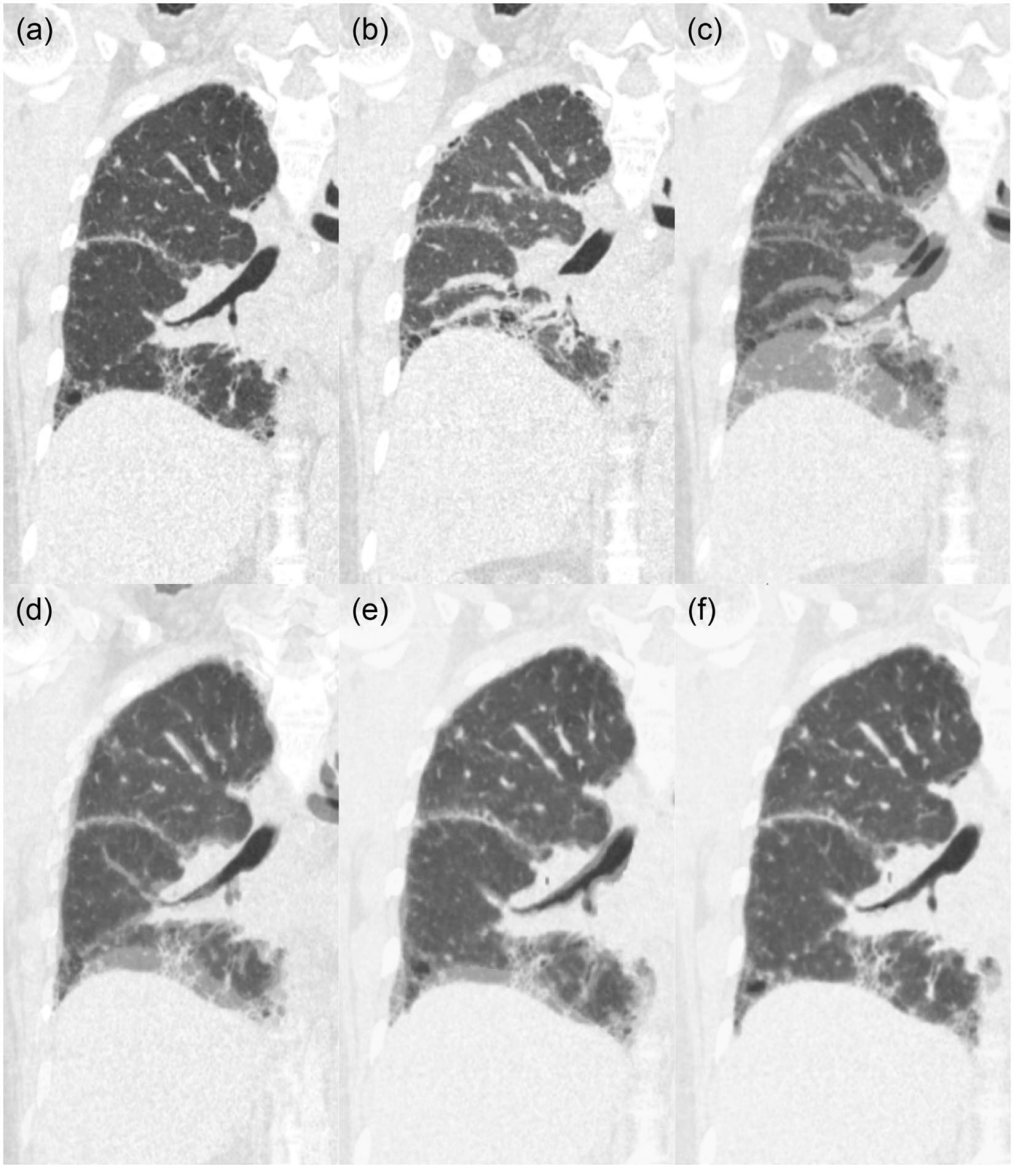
Sequential registration processes for a precise image registration of paired (a) full-inspiratory and (b) full-expiratory CT images. (c–f) Overlay images shows sequential inflations of a segmented lung in expiratory CT images to align with a segmented lung in inspiratory CT images: (c) before alignment, (d) after a surface-based affine registration, (e) landmark-based registration, and (f) attenuation-based deformable registration.
